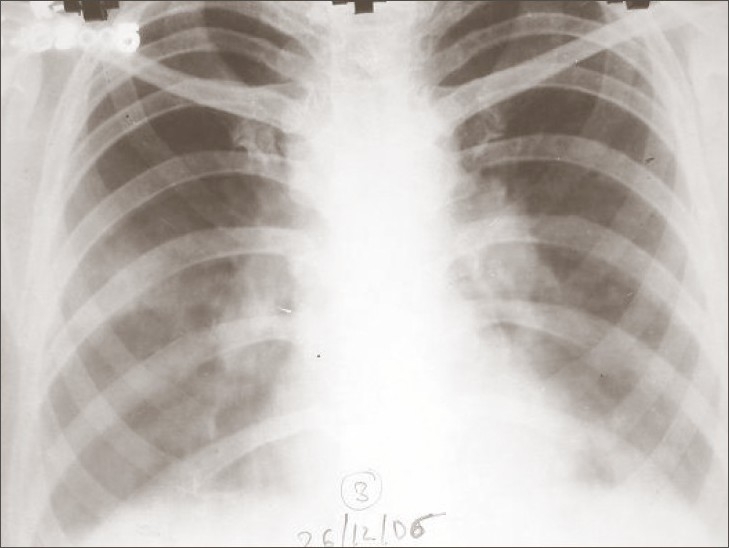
Chest X-ray revealed bilateral hilar prominence with left-sided pleural effusion
